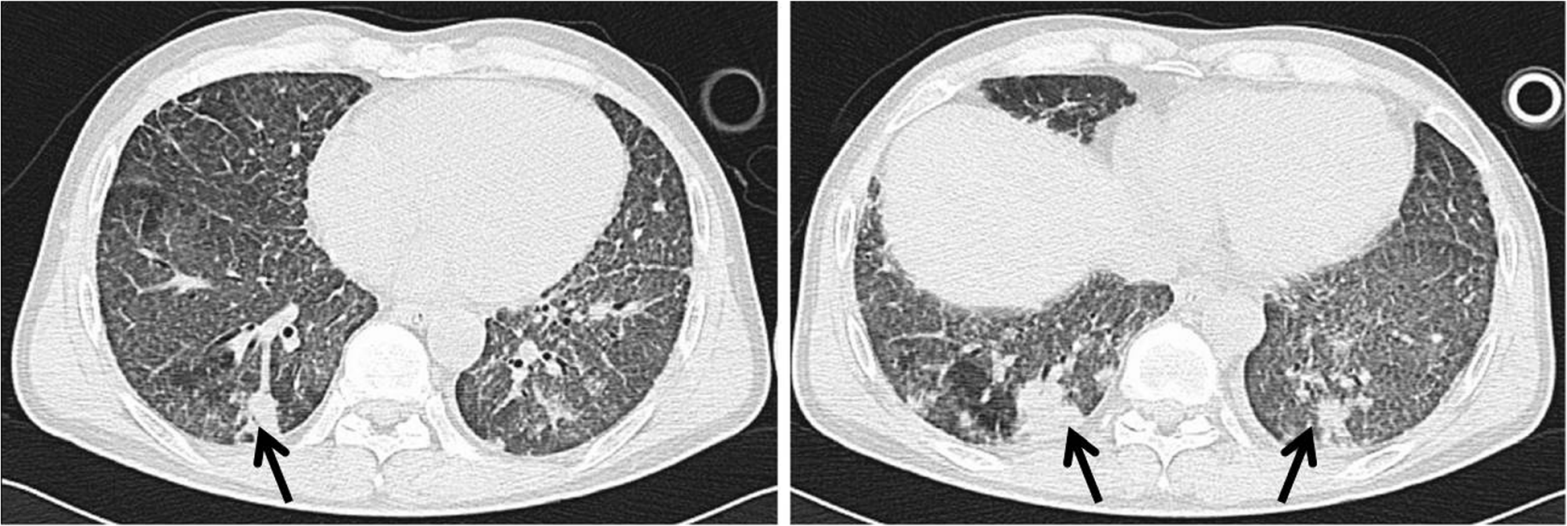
Chest CT image performed one day after the whole lung lavage showing newly multi-nodular consolidations (black arrow) and patchy opacities in both posterior segments of lower lobes.
